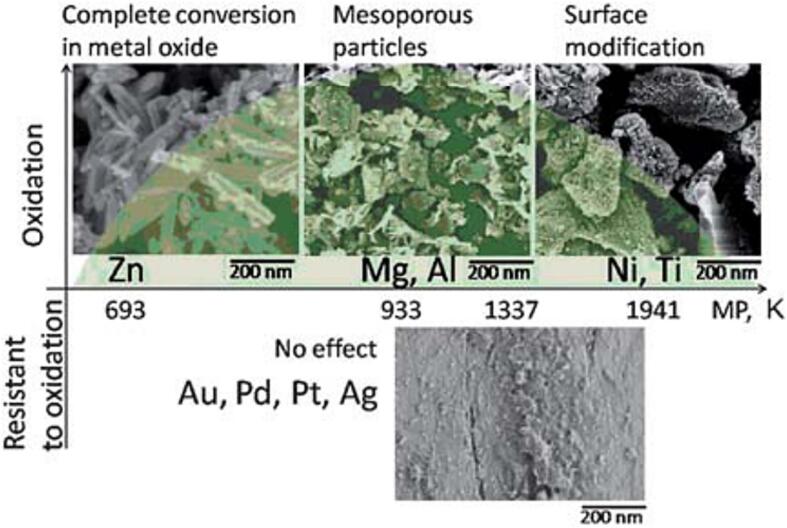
Ultrasonic exposure of various metals in which no effect was observed for metals resistant to oxidation and modification of metal particles, which are able to oxidize, was dependent on their melting point. Reproduced with permission of [145]. Copyright 2010 The Royal Society of Chemistry.
